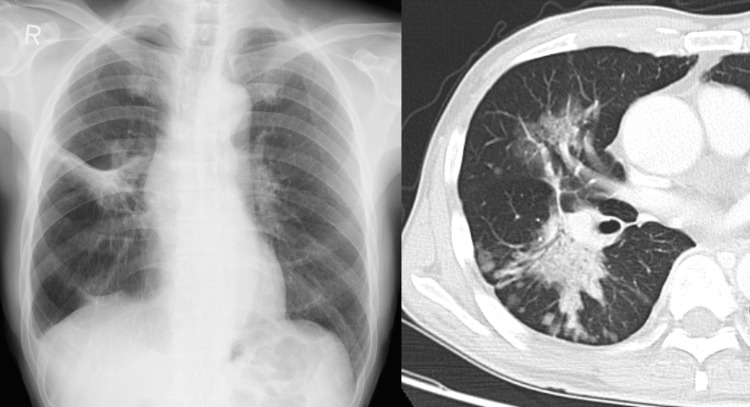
Chest radiography and CT images on admission Ground-glass opacity on the right middle lung lobe and consolidation on the right lower lung lobe with multiple patchy ground-glass opacities in its periphery were observed.
